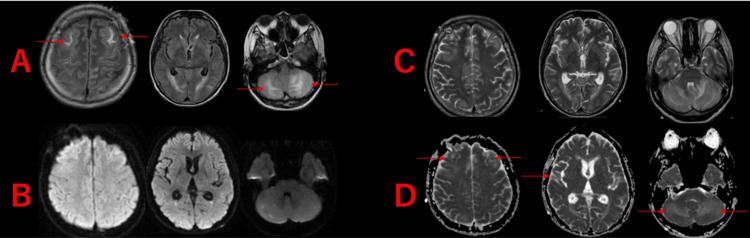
Brain Magnetic Resonance Imaging (MRI) on the Sixth Day of Hospitalization A: Fluid-attenuated inversion recovery (FLAIR), B: Diffusion-weighted imaging (DWI), C: T2-weighted imaging (T2WI), D: Apparent diffusion coefficient (ADC) High-signal areas observed on FLAIR and ADC at the time of admission have improved (red arrow).
